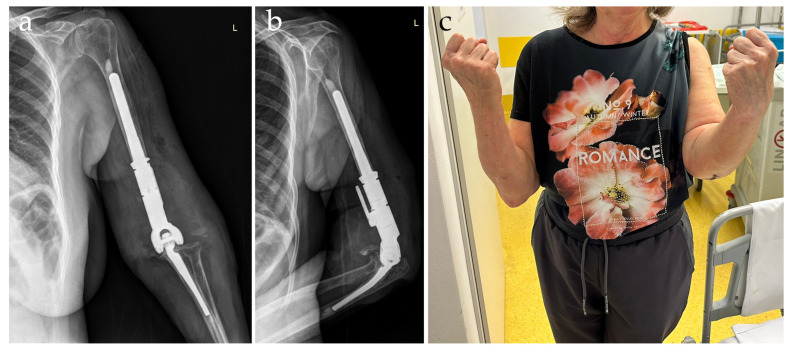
Postoperative radiographic images taken the next day after surgery in anteroposterior (a) and lateral (b) views. The patient underwent an intensive recovery protocol under permanent analgesia, minimizing the administration of opioids, with a single dose of morphine administered postoperatively. Mobilization exercises were encouraged on the first day after surgery, leading to the recovery of elbow flexion of more than 90° after the first 3 days (c). Following surgery, the patient was instructed to start an intensive physical therapy program, to not lift objects heavier than 3 kg, perform proper wound cleaning, and apply ice twice per day for 20 min for the first two weeks. Her recovery was quick, and the patient recovered her functional status by 4 weeks. One month after the total elbow arthroplasty, the patient achieved a flexion range of 110–115 degrees, full extension, and supination of 70–80 degrees.
